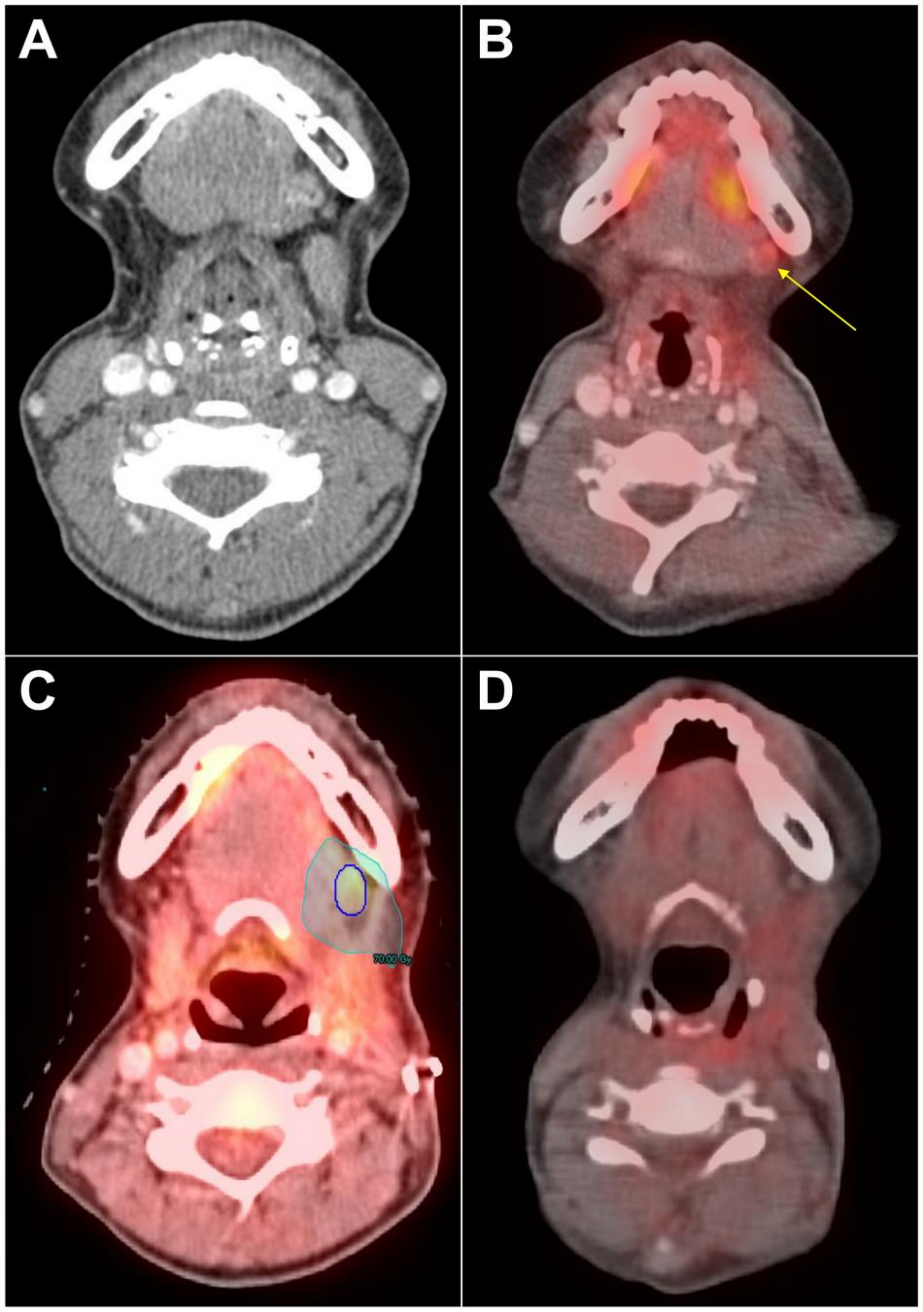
Representative images of a patient who had a management change because of postoperative FDG-PET/CT findings. This patient presented with a left lateral tongue nodule, biopsy of which showed squamous cell carcinoma. Preoperative CT neck and chest with contrast (A) showed bilateral, nonspecific neck lymph nodes all <1 cm in short-axis and no gross oral cavity lesion, and neck ultrasound did not find any abnormal lymph nodes. They underwent left partial glossectomy and left neck dissection which showed a 1.5 cm tumor with 0.9 cm depth of invasion, 2/21 lymph nodes positive without extracapsular extension, overall AJCC 8th stage pT2N2b. Additionally, margins were negative, and there was no perineural or lymphovascular invasion. At radiation oncology consult, adjuvant radiotherapy to a maximum dose of 60 Gy without concurrent systemic therapy was recommended, as was FDG-PET/CT, which showed a new, enlarged, FDG-avid left submandibular lymph node (B). Subsequent biopsy showed squamous cell carcinoma. Repeat surgery was attempted but the node was unable to be localized intraoperatively, so the patient proceeded with adjuvant radiotherapy to a maximum dose of 70 Gy (C) with concurrent cisplatin 100 mg/m^2^ every 3 weeks. Posttreatment FDG-PET/CT showed resolution of the lymph node (D).
